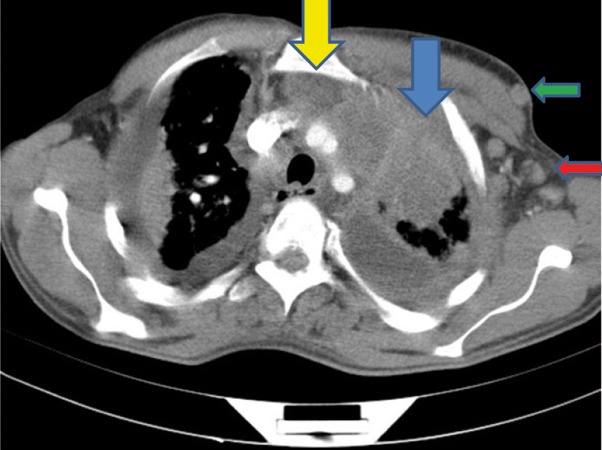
A, Contrtast-enhanced CT (CECT) of the thorax shows left axillary lymphadenopathy (red arrow), subcutaneous nodule anterior to the left pectoralis major (green arrow), left upper lobe mass with collapse/consolidation (blue arrow) and anterior mediastinal adenopathy (yellow arrow).
